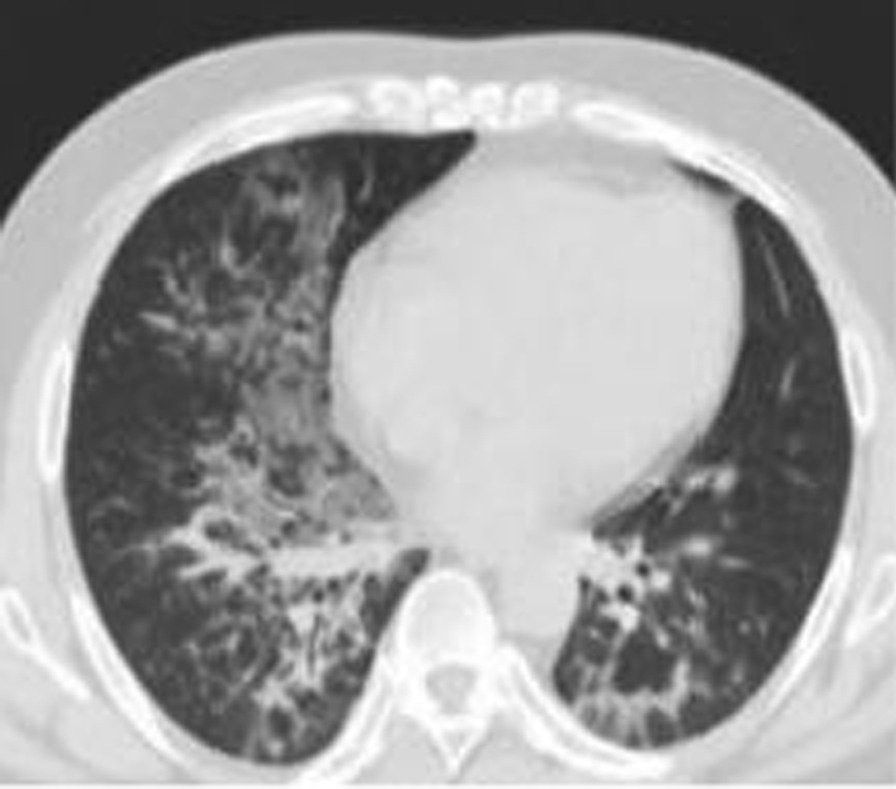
2020–5-30 chest CT scan revealing patchy ground glass opacities in the lungs. Patchy ground glass opacities are mainly located in the inner field, and distributed along the bronchi
